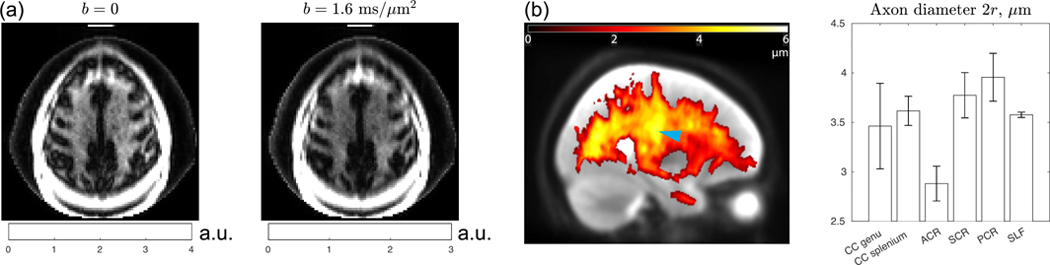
Myelin water selected diffusion MRI measurements and axon diameter mapping in brain white matter. To selectively measure myelin water diffusion signals, we performed diffusion MRI with inversion recovery and short repetition time to saturate signal contributions from (intra- and extra-) axonal water and cerebrospinal fluid. (a) The non-diffusion-weighted-image averaged over 68 repetitions (left) and spherical mean signal averaged over 80 directions at b-value = 1.6 ms/*μ*m^2^ (right) showed negligible partial volume effects from cerebrospinal fluid. The skull fat signal was noticeable due to incomplete fat saturation in images with low SNR. (b) Fitting MyCaliber model (9) and (6) to normalized spherical mean signal in white matter, we estimated axon diameter maps averaged over 3 healthy subjects (left) and showed thick axons in the corticospinal tract (blue arrow). The ROI analysis in JHU DTI white matter atlas (right) showed an anterior-to-posterior gradient (from ACR, SCR, to PCR) of axon diameters in the brain. The a.u. indicated the arbitrary units of the MRI signal. The error bar indicated the standard deviation of 3 subjects in each ROI. CC = corpus callosum; ACR, SCR, PCR = anterior, superior, and posterior corona radiata; SLF = superior longitudinal fasciculus.
